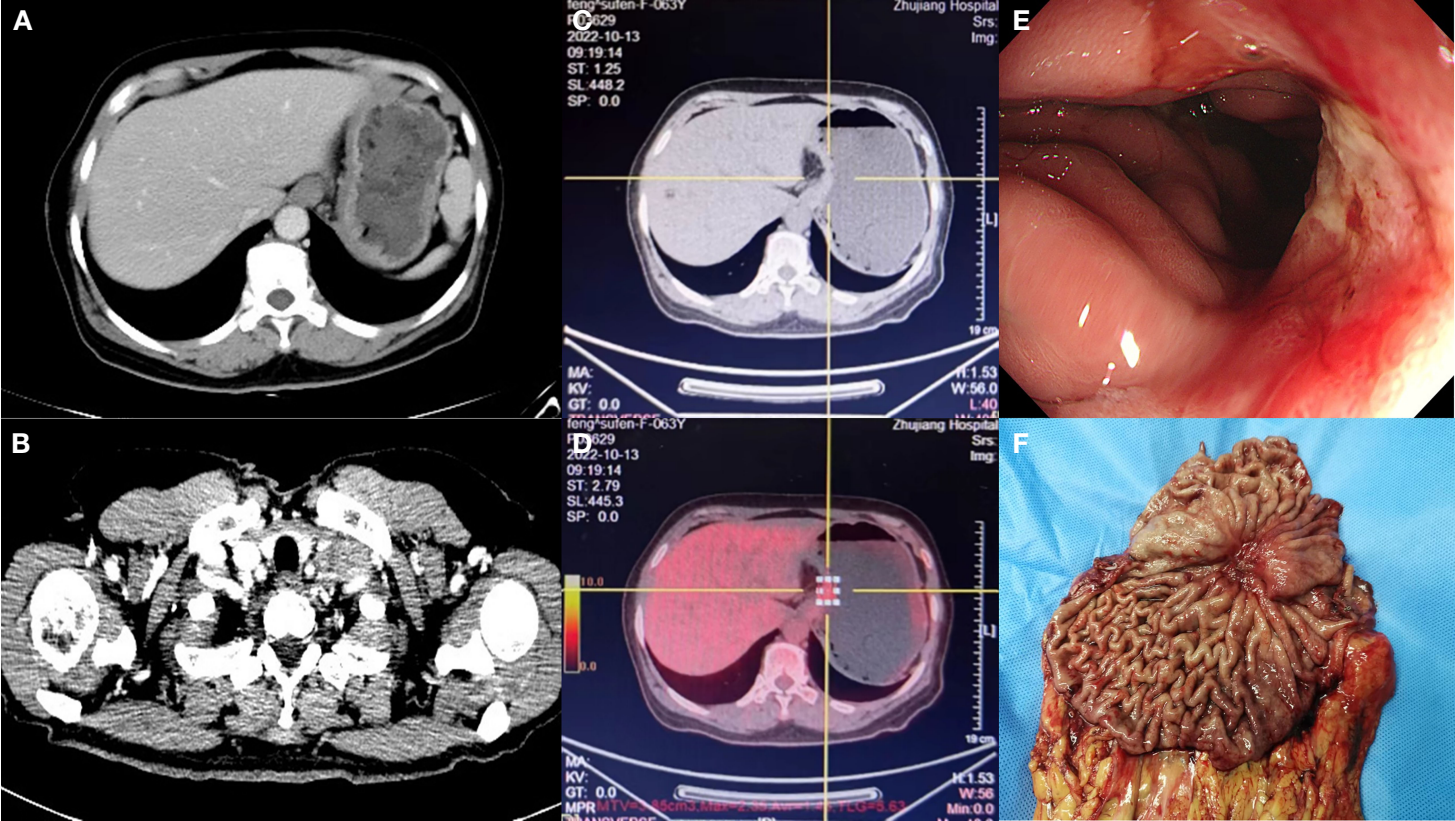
Preoperative contrast-enhanced computed tomography (CT) showed that the uneven thickening of the gastric wall and multiple enlarged lymphatic in the left cervical was reduced (A, B). The positron emission tomography (PET)-CT showed that the previously uneven thickening of gastric wall and the previously metabolically active lymph nodes in the left clavicular region was reduced, and metabolism dropped to the background (C, D). Gastroscopy after treatment revealed an ulcerated mass (E). The primary focus of stomach (F).
